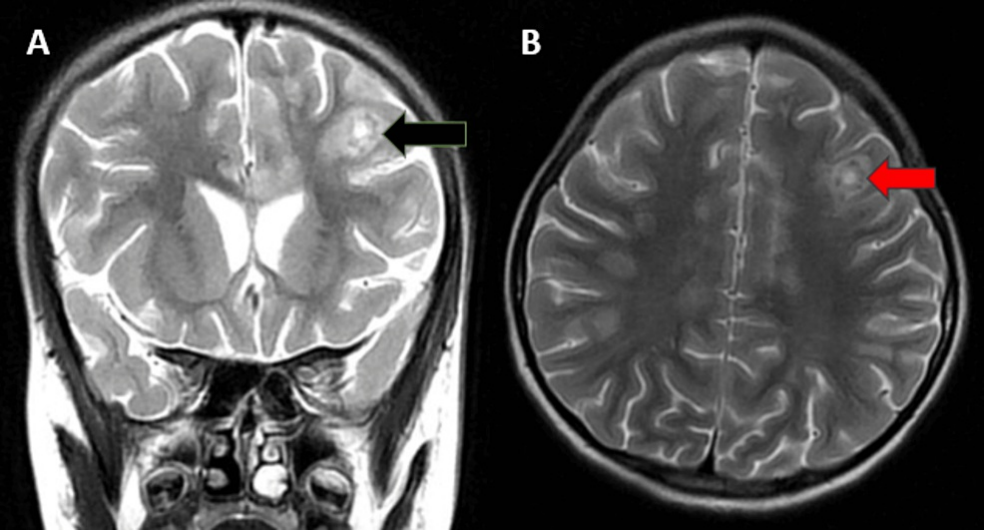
Coronal T2 (A) and axial T2 (B)-weighted MRI showing hyperintensities in the subcortical white matter. MRI: magnetic resonance imaging
